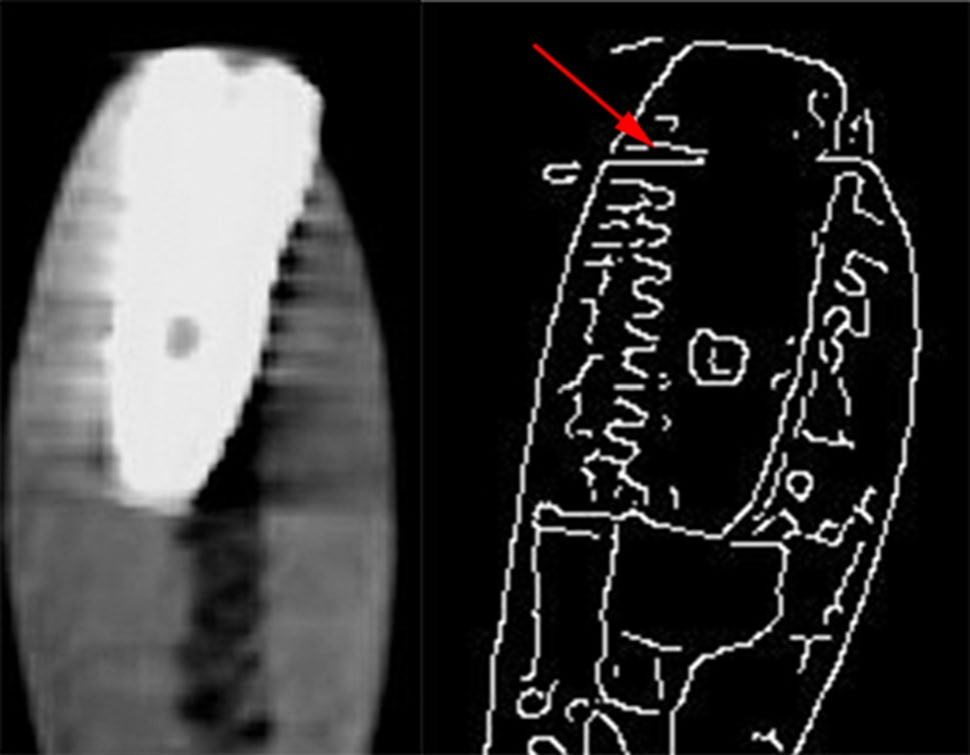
CBCT section of a fractured implant and its related Canny image (Arrow points to the fracture area which cannot be detected on the original image).
